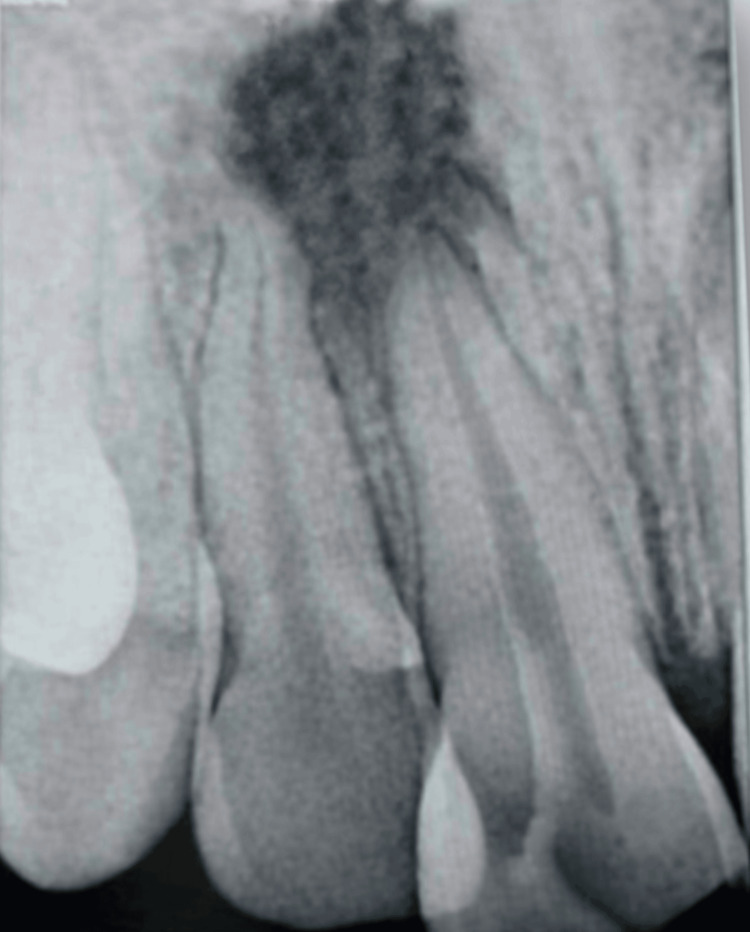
Radiographic examination
